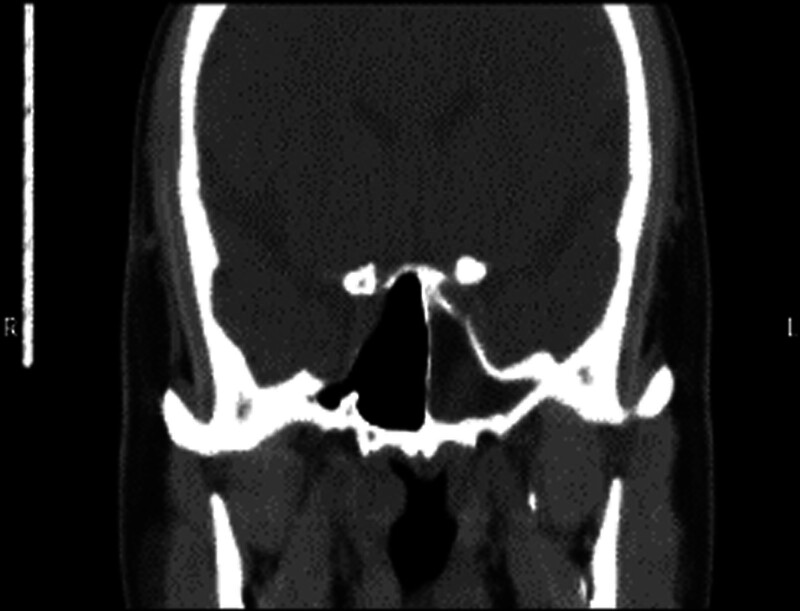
A 49-year old male patient with preoperative sinus CT coronal view. CT = computed tomography.
